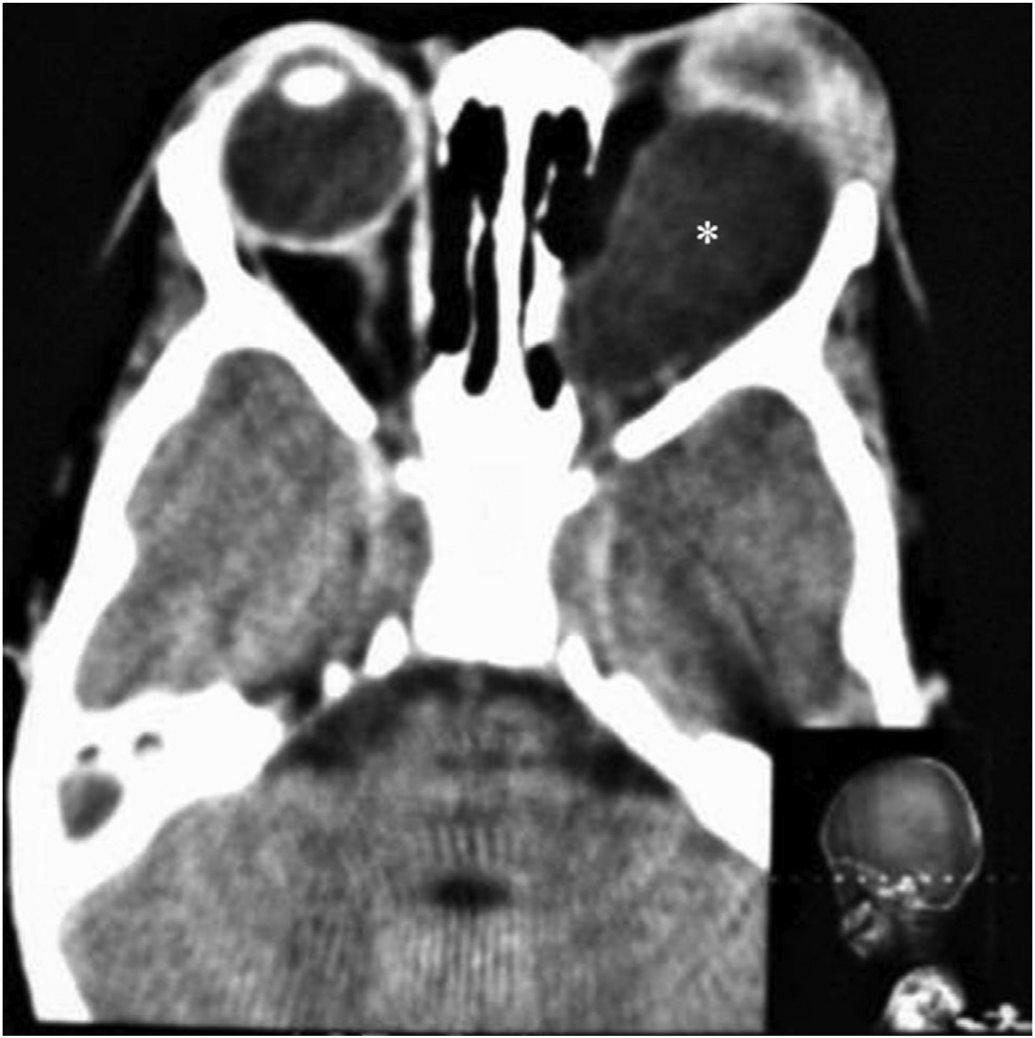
Axial orbital CT scan in a 2-year-old child shows a well-defined hydatid cyst (Asterisk) in the intraconal space of the left orbit. Note the anterior displacement of globe in the involved eye. (Photos are from our own patient(s) and they had not been published previously elsewhere.).
